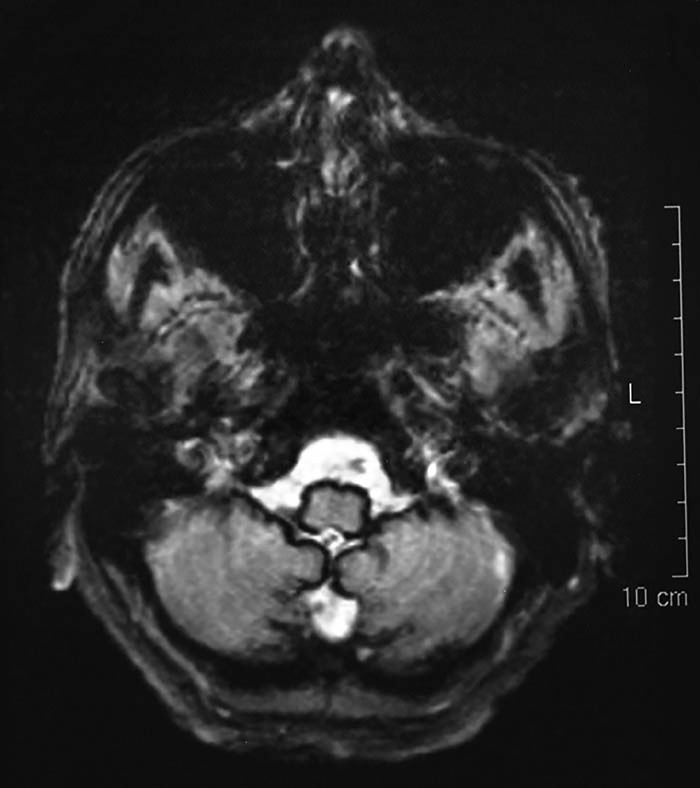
Axial T2-weighted MRI of the brain, showing haemosiderin deposition around the medulla and cerebellum, in a case of superficial siderosis. (Gradient echo (GRE)/T2* imaging would have demonstrated these changes more vividly.)
